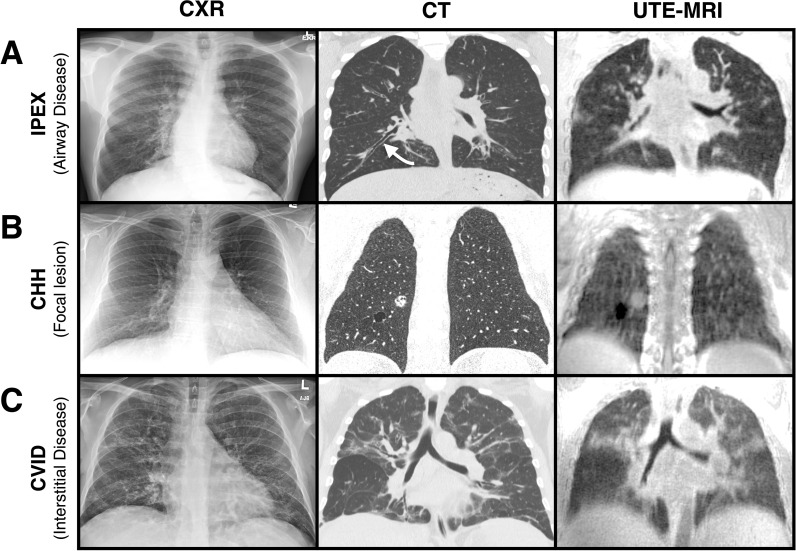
Radiologic manifestations of inborn errors of immunity across pulmonary imaging modalities. Radiologic manifestations in three distinct inborn errors of immunity (IEI) are shown across complementary pulmonary imaging modalities: chest radiograph (CXR), computed tomography (CT), and ultra-short echo time magnetic resonance imaging (UTE-MRI). Radiographic findings (A) Immunodysregulation polyendocrinopathy enteropathy X-linked (IPEX) syndrome: peripheral airway plugging and bronchiectasis in the posteromedial right lower lobe (indicated by white arrow); (B) cartilage-hair hypoplasia (CHH): a 22 × 18 mm part-solid, predominantly ground-glass and cystic nodule in the right lower lobe; (C) common variable immunodeficiency (CVID): stable bilateral peribronchovascular and peripheral reticulation, interstitial thickening, and architectural distortion.
